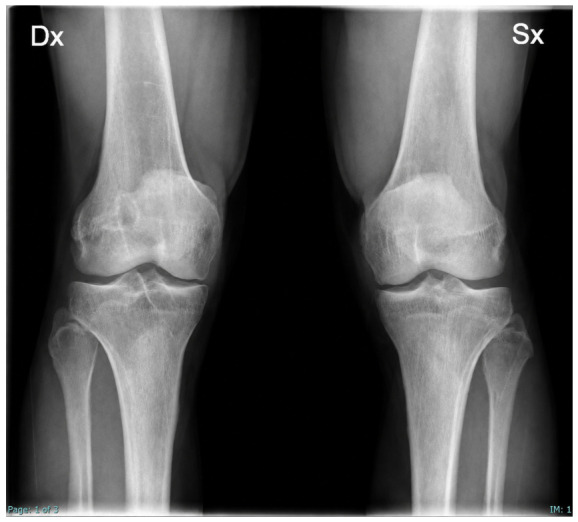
Radiograph of both knees demonstrating Kellgren–Lawrence grade 2 osteoarthritis, characterized by definite osteophyte formation, early joint space narrowing, and subchondral sclerosis of the tibial plateaus.
